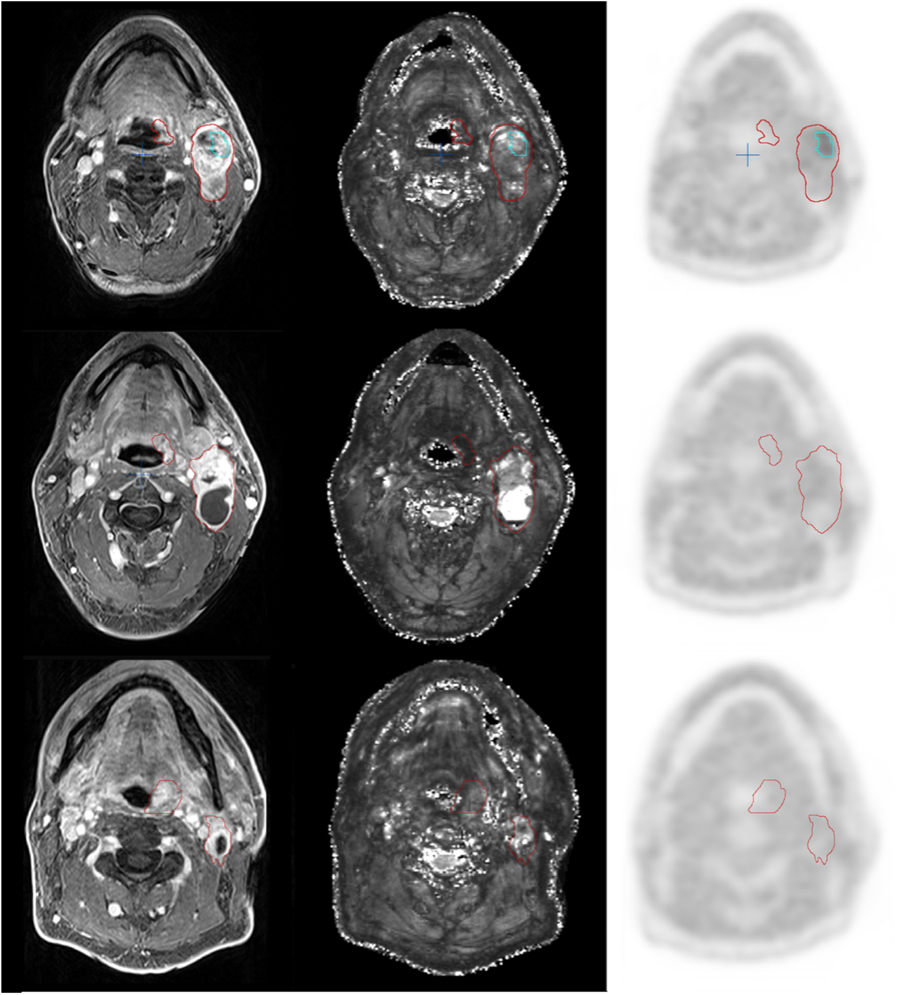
Representative example of imaging modalities MRI T1, T2*, and FMISO-PET. Primary tumour and lymph node metastasis (pt. 5, tonsillar carcinoma) at week 0, 2, and 5 (upper, middle, lower panel): co-registered image sets from MRI T1, MRI T2*, FMISO-PET (left to right). Red contours: GTV-T, GTV-LN. Blue contour: HSV-LN
